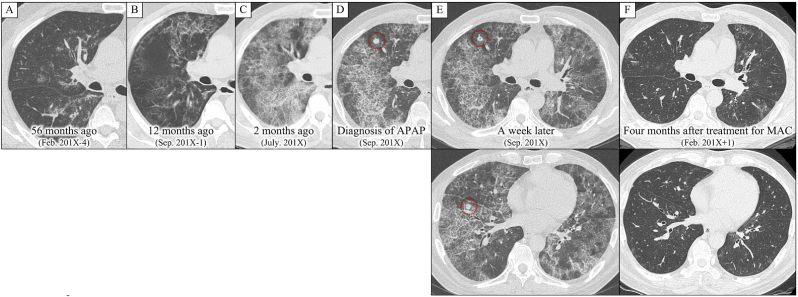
Chest computed tomography (CT) on the first visit to a nearby hospital demonstrated patchy bilateral ground-glass attenuation (GGA) (A). The patient was followed-up on suspicion of interstitial pneumonia, and the GGA in both lungs worsened gradually (B-D). Chest CT at the time of diagnosis of autoimmune pulmonary alveolar proteinosis demonstrated nodules in both lungs (dotted area) (D), and cavities were visible within the nodules after 1 week (dotted area) (E). After a 6-month treatment with rifampicin, ethambutol, and clarithromycin, the GGA and nodules were improved remarkably (F). APAP, autoimmune pulmonary alveolar proteinosis; MAC, *Mycobacterium avium* complex.
